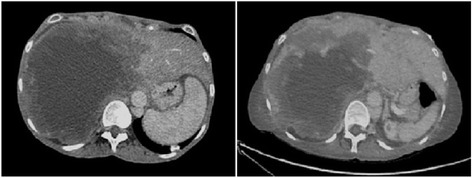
Abdominal computed tomography scan showing hepatomegaly with a 15 cm cystic mass in the right lobe with finely lobulated contours, and perilesional calcifications. The lesion extended into the left lobe of the liver, infiltrating the hepatic hilum, and provoking intrahepatic bile ducts dilatation, compression of the inferior cava vein and the left branch of the portal vein, and occlusion of the right portal vein
